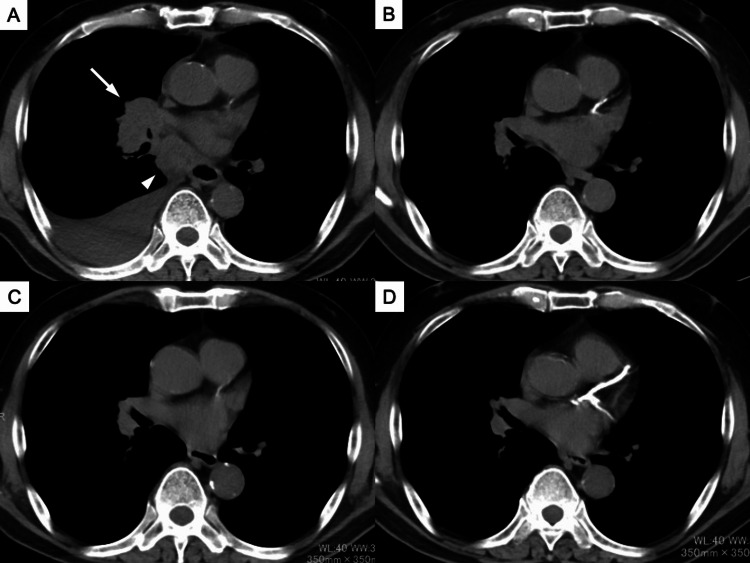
Chest CT shows the primary tumor in the right middle lobe (white arrow), mediastinal lymphadenopathy (white arrowhead), and right pleural effusion at the time of lung cancer diagnosis (A). The primary tumor and mediastinal lymphadenopathy have shrunk, and the right pleural effusion has disappeared after four cycles of combination therapy with atezolizumab plus carboplatin and etoposide (B). Each lesion remains decreased in size or has disappeared during seven cycles of maintenance atezolizumab therapy (C), and even 18 months after the discontinuation of atezolizumab treatment (D).
